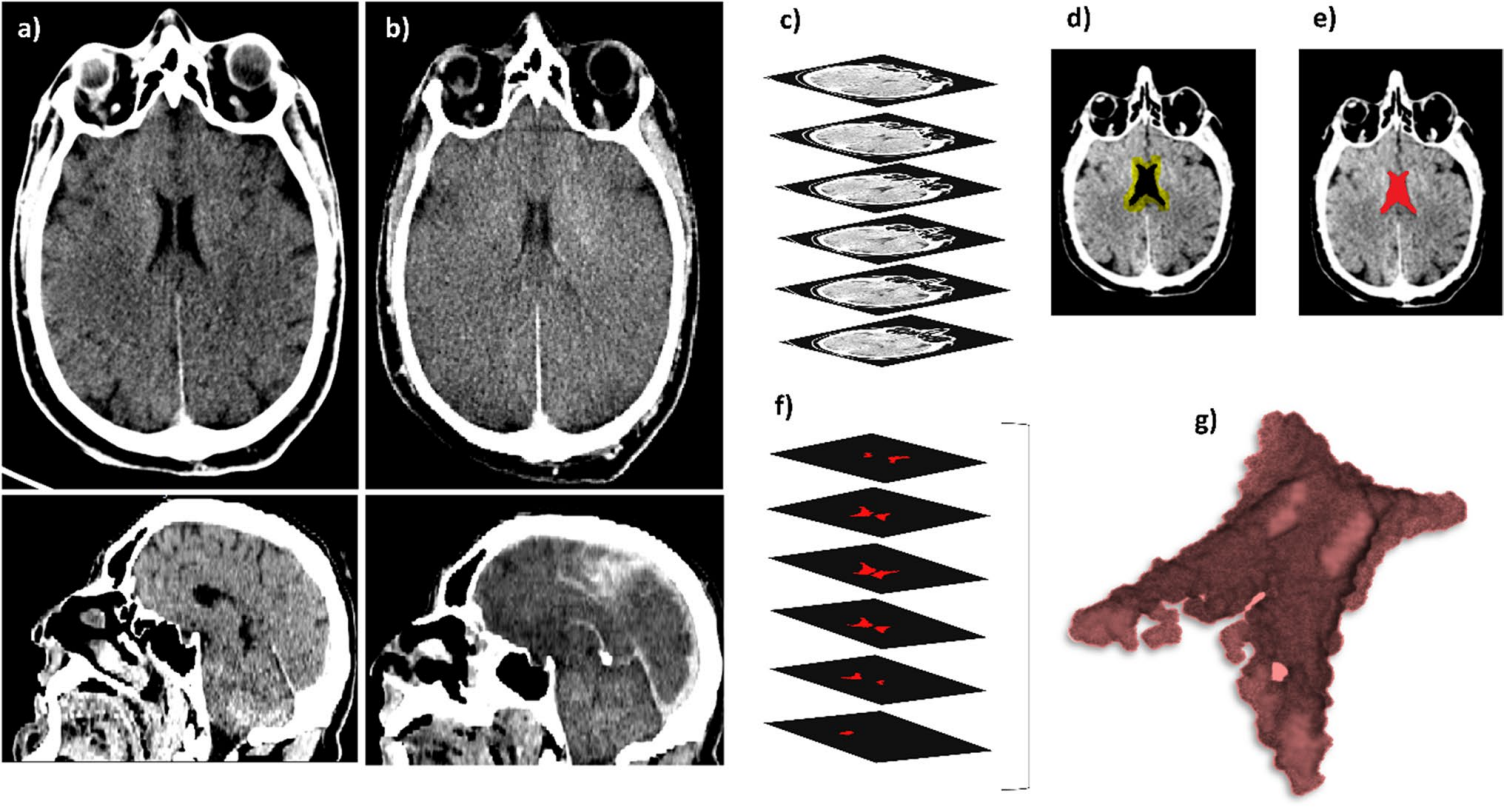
PMCT images in the axial (upper) and in the sagittal (lower) planes of the same representative brain slice at different times after death, namely 24 h (a) and 114 h (b). In c, a graphical representation of the input image set for the segmentation algorithm is shown; for each selected image, a contour (d) and a mask (e) of the ventricle region for each CT slice are shown. The extrapolated masks of the ventricle slices are represented (f), and three-dimensional reconstruction of the cerebral lateral ventricle is shown (g)
